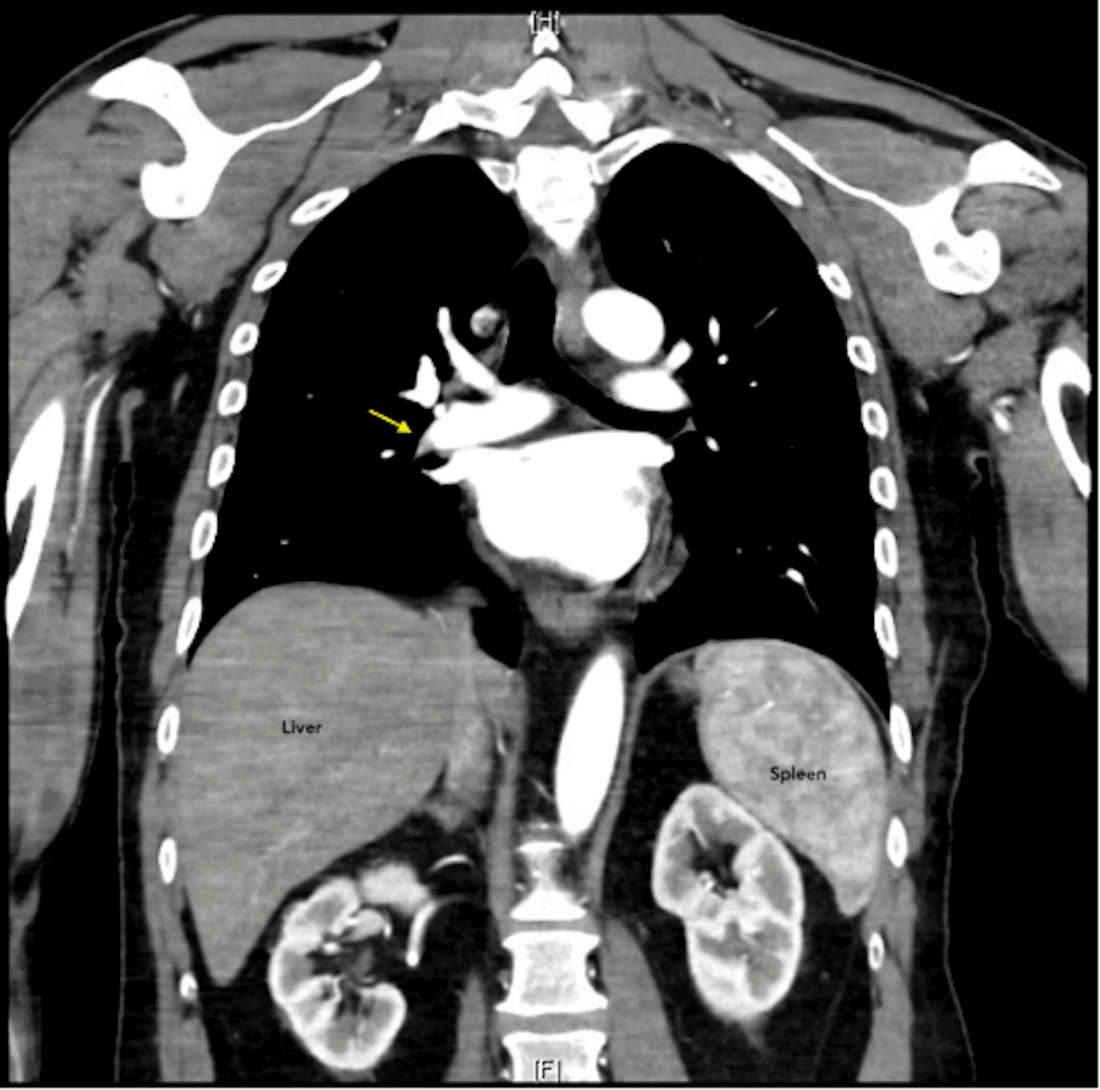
Chest tomography angiogram (CTA) demonstrating a filling defect consistent with a pulmonary embolism (arrow)
